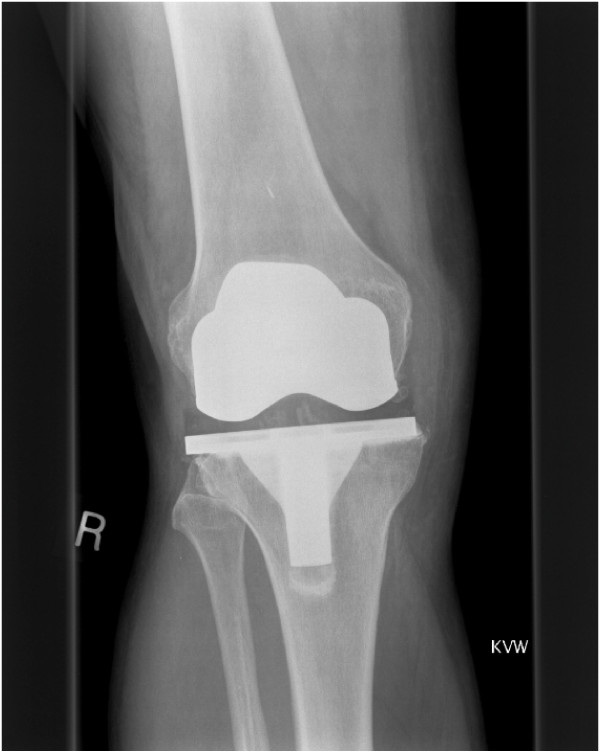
Radiograph at 5 years post-total knee arthroplasty, with medial femoral osteophyte (arrow) and tibial component overhang compared to femoral component.
